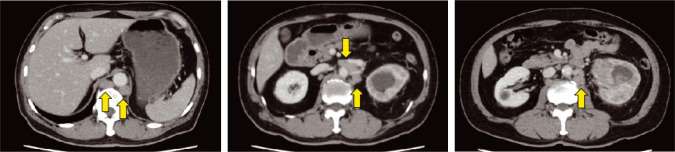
Computed tomography at the first visit. A moderately enhanced mass occupies the left renal pelvis, accompanying hydronephrosis and multiple para-aortic lymphadenopathy (arrows).
